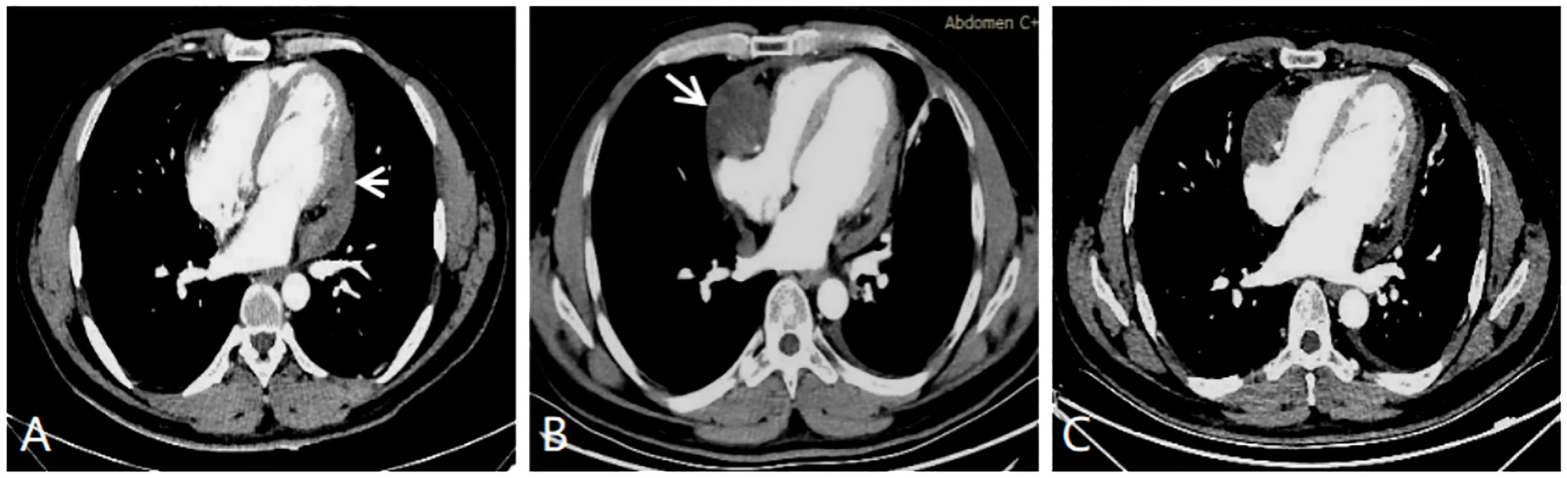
(A) CT enhanced image after six cycles of chemotherapy showing that the tumor has significantly regressed *(white short arrow).*
